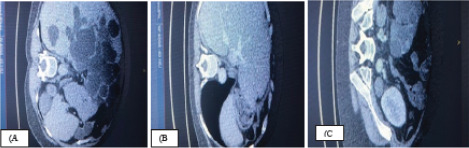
CT scan showing cysts before liver transplantation, B) after simultaneous liver and, C) renal transplantation (RT in the left iliac fossa).
